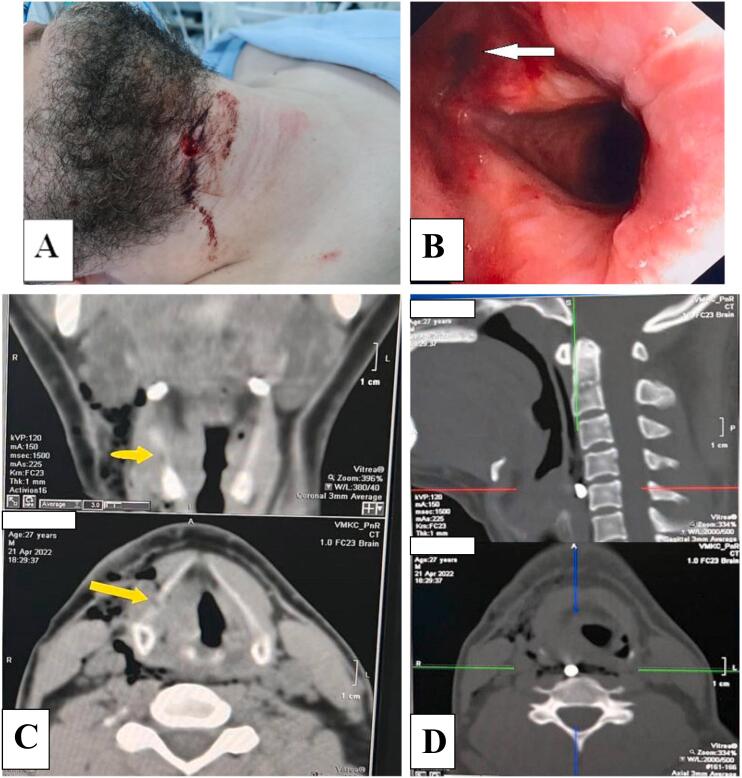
Examination of a Gunshot Wound to the Laryngopharynx (A- view of the wound on admission; B - endoscopic examination - injury of the right lateral surface of the pharynx; C - CT scan - the site of injury of the right half of the thyroid cartilage; D - localization of a foreign body, metal fragment).
